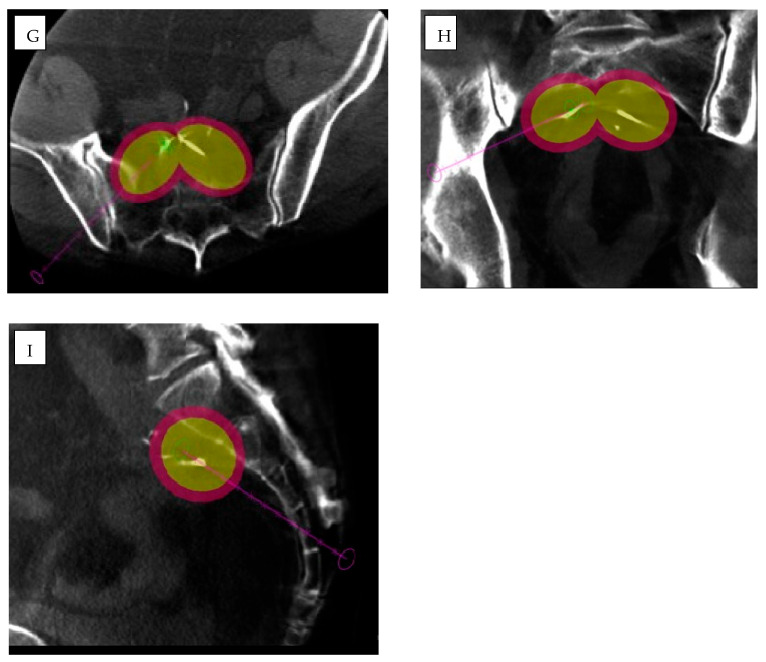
A 61-year-old man with rectal adenocarcinoma previously treated with surgery, adjuvant chemotherapy, and radiotherapy. Pre-procedural MRI, including axial (A) and sagittal (B) T2-weighted images, (C) T1-weighted post-contrast image, and (D) diffusion-weighted imaging (DWI) with (E) apparent diffusion coefficient (ADC) map, together with (F) 18F-FDG PET/CT, demonstrate a heterogeneous mass in the presacral region consistent with local disease recurrence (red arrows). (G–I) Intra-procedural cone-beam CT (CBCT) in axial (G), coronal (H), and sagittal (I) planes obtained using XperGuide software (Philips Healthcare) for cryoablation probe placement (purple arrows), and XperCT (Philips Healthcare) for ablation zone prediction (purple and yellow circles).
